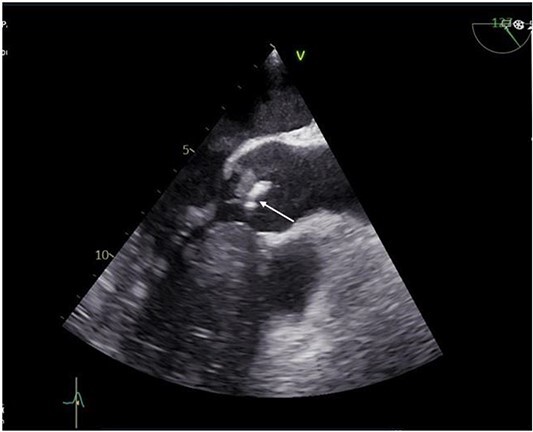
Preoperative transthoracic echocardiogram (TTE) showing vegetation on the aortic valve.
